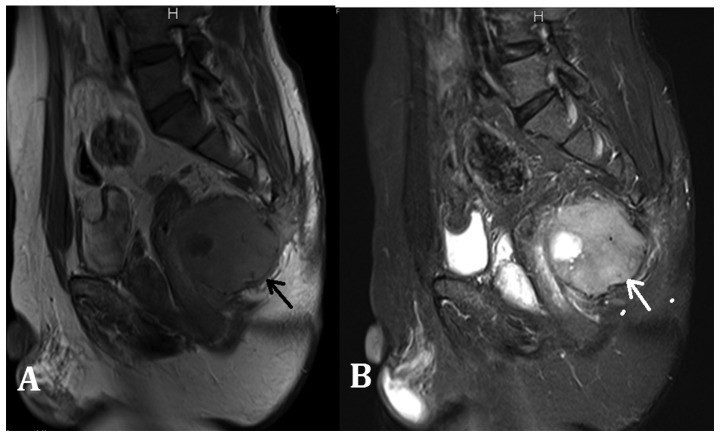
Nine months after the first surgery, a follow-up magnetic resonance imaging scan (A, T1; B, T2) showed a recurrent mass at the site of the original giant cell tumor of the bone.
